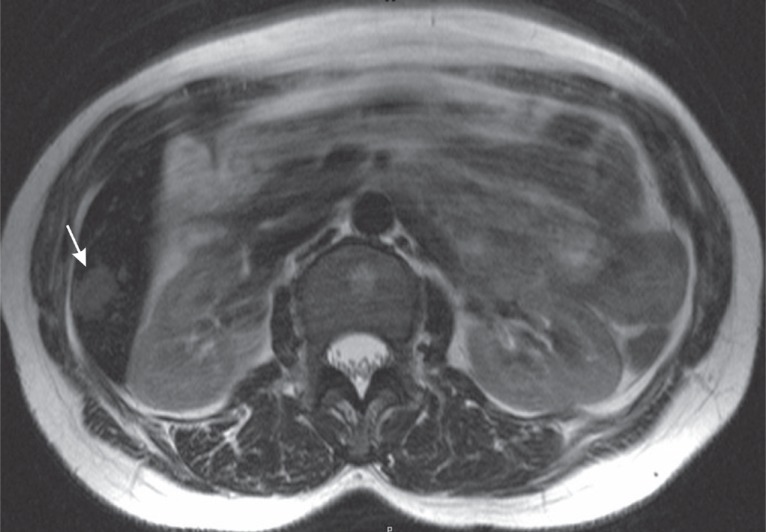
Axial T2 weighed MRI sequence. The hepatic hemangioma has an atypical T2 signal intensity (arrow) which is not as bright as cerebrospinal fluid as usually found in hepatic hemangiomas.
